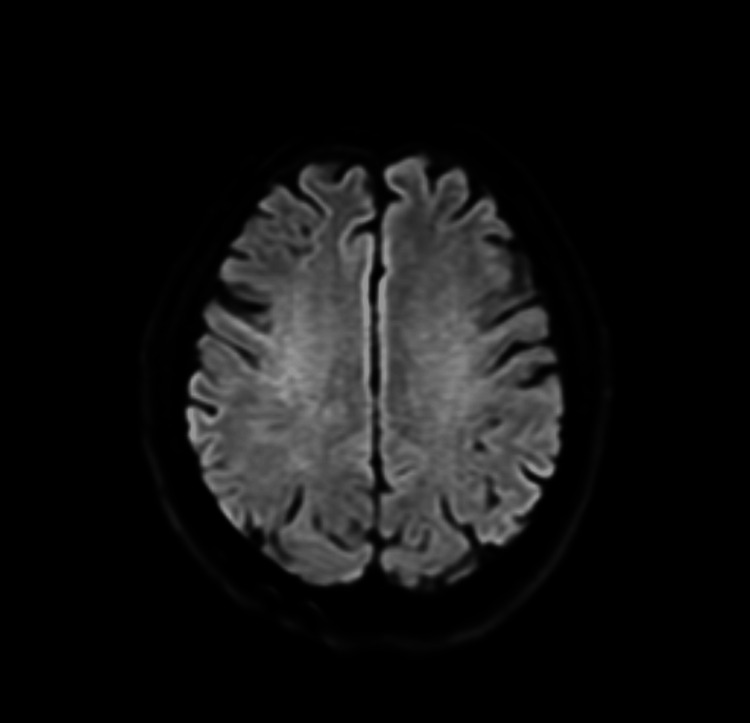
Axial diffusion-weighted MRI of the brain at the level of the centrum semiovale demonstrating no diffusion restriction
